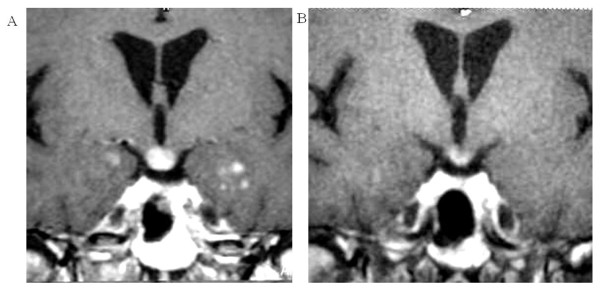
Partial response to vinblastine chemotherapy. Hypothalamic and temporal lesions. Coronal T1-weighted magnetic resonance imaging (MRI) with gadolinium before treatment with vinblastine (A) and 12 months after treatment initiation, showing a partial response (B).
